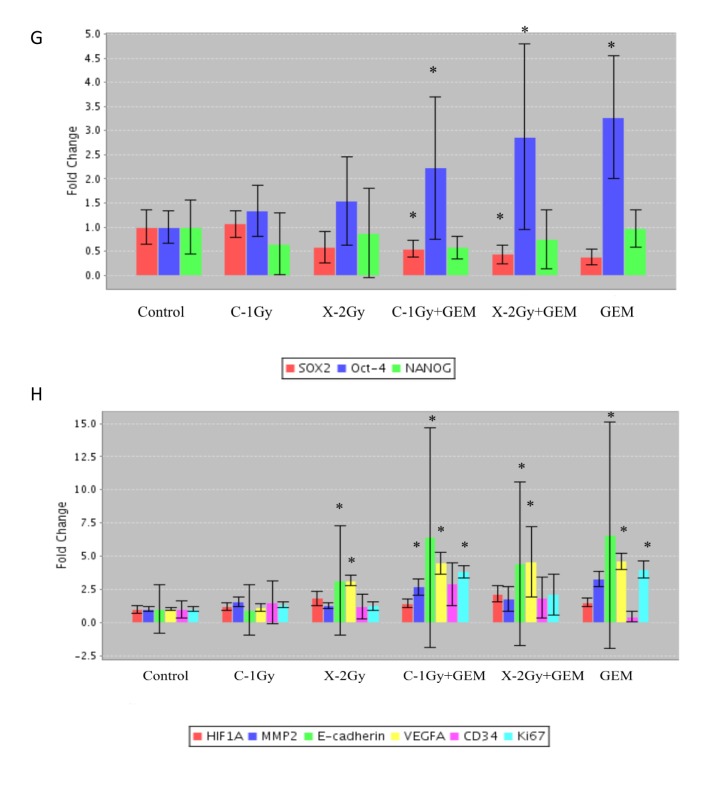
(A) Morphological changes of PK45 cells at 72 h after treatment with carbon ion beam alone, X-ray alone or in combination with gemcitabine (GEM). (B) Representative photos and quantification of apoptosis in CD44+/ESA+ cells and CD44−/ESA− cells 48 h after carbon ion beam alone, X-ray alone or in combination with gemcitabine (GEM) in PK45 cells. (C) Clustergram of Custom RT-PCR Array of PK45 cells 72 h after carbon ion beam alone, X-ray alone or in combination with 10 nM gemcitabine.9GEM) Expression changes of apoptosis and autophagy (D) senescence (or cell cycle) (E), DNA repair (F), stemness angiogenesis and metastasis-related genes after carbon ion beam alone, X-ray alone or in combination with gemcitabine 9GEM) in PK45 cells. *. *p* < 0.01 compared to control.
